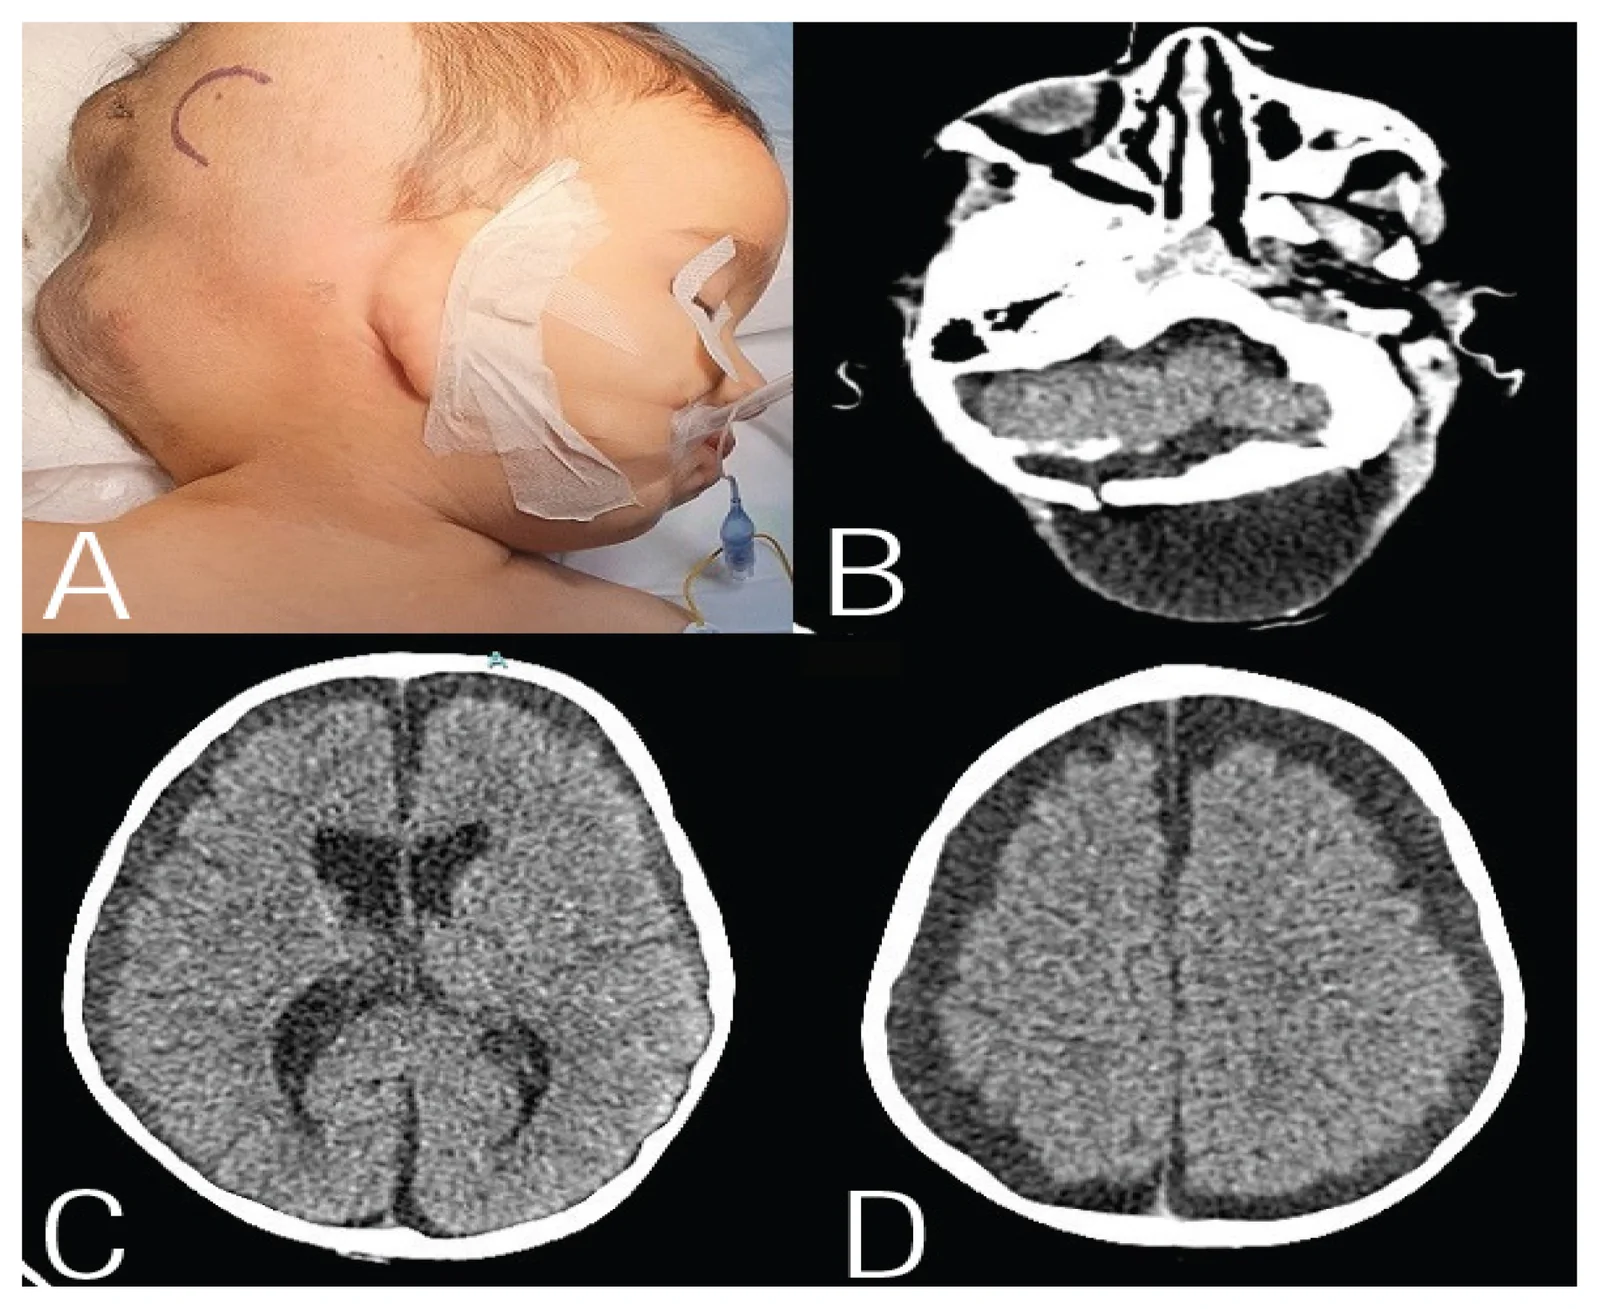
A: Photograph showing a tense pseudomeningocele at the external ventricular drain site (arrow down) and surgical site (arrow up) immediately before shunt surgery as well as the shunt incision mark (asterisk). B: An axial view of a computed tomography (CT) scan of the brain showing the pseudomeningocele (asterisk). C & D: A CT of the brain showing the progression of subdural hygroma and further regression of hydrocephalus.
